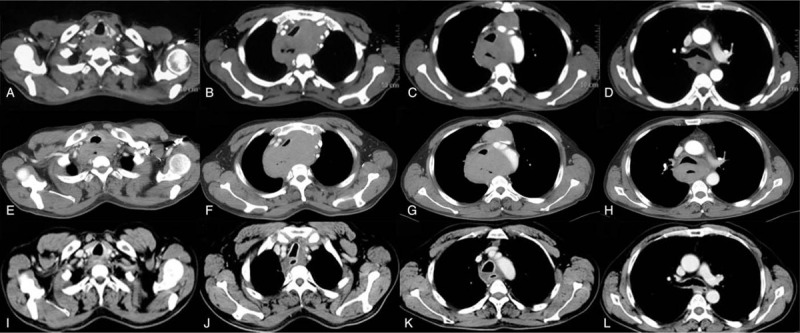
CT images of patients before and after treatment. A–D: the baseline CT of the patient; E-H: disease progression after 2 cycles CHOP regimen; I–L: the esophageal lesions disappeared after radiotherapy. CHOP = cyclophosphamide–Adriamycin–vincristine–prednisone.
